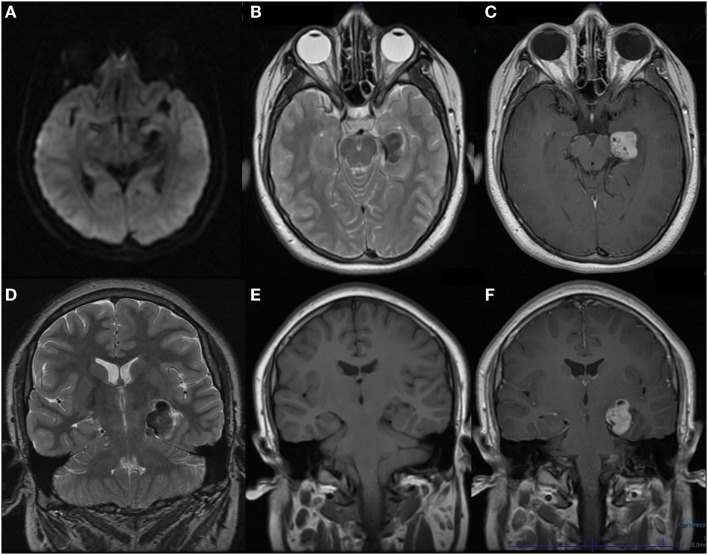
MRI brain with and without contrast obtained at onset of seizures demonstrating left mesial temporal lobe lesion. (A) Axial DWI (B) Axial T2 (C) Axial T1 post-contrast (D) Coronal T2 (E) Coronal T1 pre-contrast (F) Coronal T1 post-contrast.
